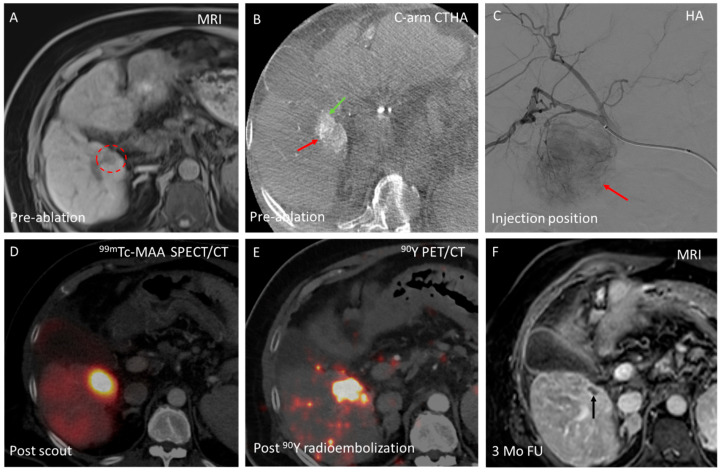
Example of conversion from an initially planned thermal ablation to a radioembolization procedure in a 55-year-old patient with a solitary HCC in segment 5. (A) The HCC (18 mm) (red circle) is visualized on MRI (T1 + gadolinium) 2 months before the planned ablation procedure; (B) On intraprocedural C-arm CT hepatic arteriography (C-arm CTHA), significant tumor growth (from 18 to 39 mm) was observed, with direct contact between the tumor (red arrow) and the gallbladder (green arrow), making ablation unfavorable. Consequently, the decision was made to switch to radioembolization and perform a ^99m^Tc-MAA scout procedure; (C) The tumor (red arrow) visualized on hepatic angiography (HA), with the intra-arterial catheter advanced to the right hepatic artery for injection of ^99m^Tc-MAA; (D) Post-scout ^99m^Tc-MAA SPECT/CT (on the same day) shows adequate tumor targeting; (E) Three weeks after the scout procedure, radioembolization with 570 MBq yttrium-90 (^90^Y) glass microspheres (estimated tumor absorbed dose: 500 Gy) was performed at the same injection position. ^90^Y PET/CT the following day demonstrated intense activity buildup in the target lesion, with no extrahepatic depositions; and (F) Contrast-enhanced MRI at 3-month follow-up showing complete response (black arrow).
